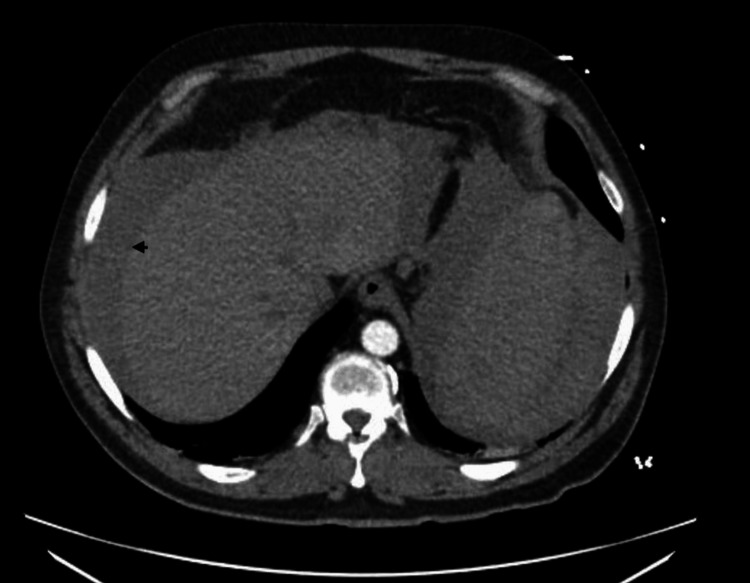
Computed tomography of the abdomen with arrow indicating moderate-to-large ascites. No lymphadenopathy, splenomegaly, cirrhosis, or features of portal hypertension were identified.
